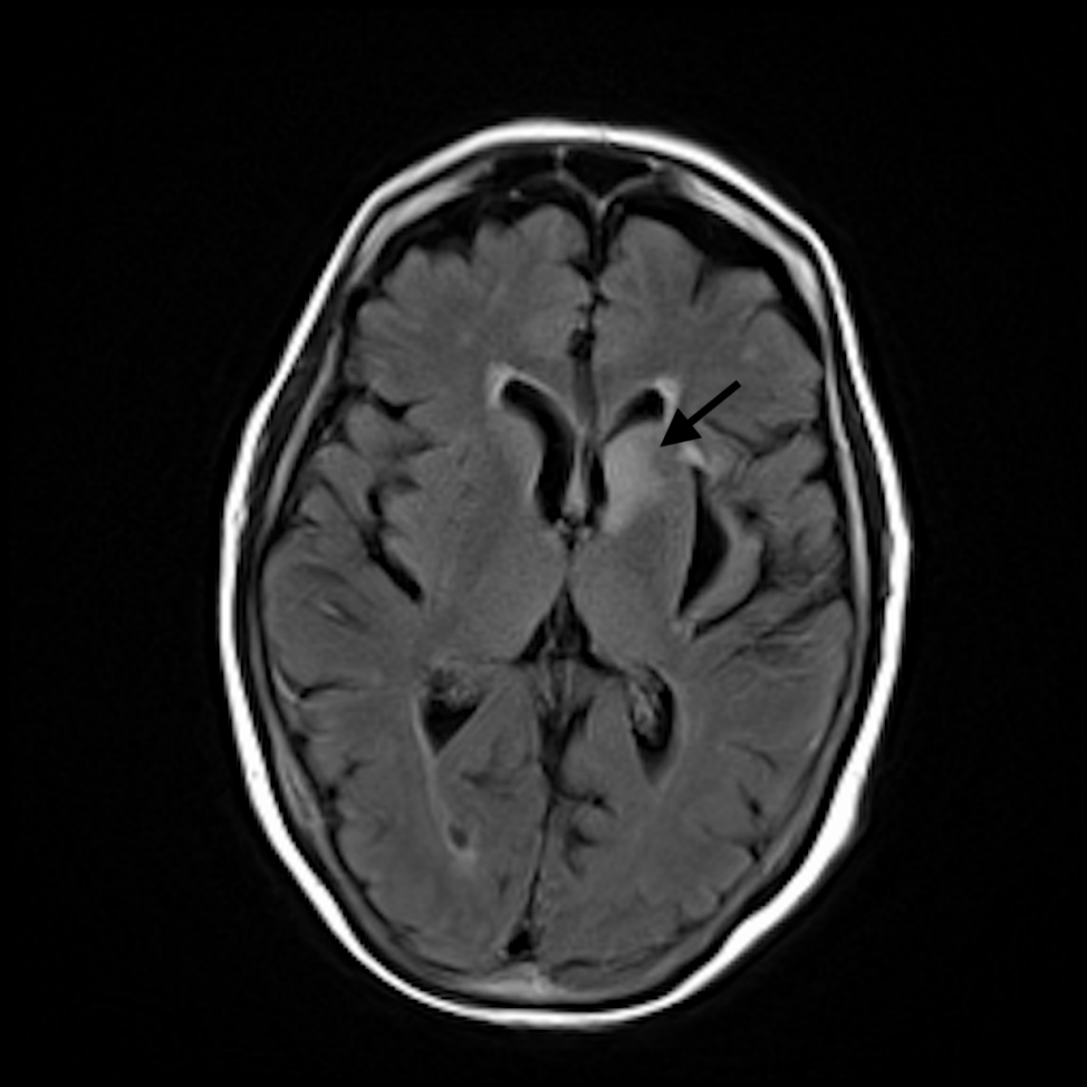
Magnetic resonance imaging (MRI) fluid-attenuated inversion recovery (FLAIR) imaging Increased signal intensity in the left caudate head on T2 FLAIR imaging.
